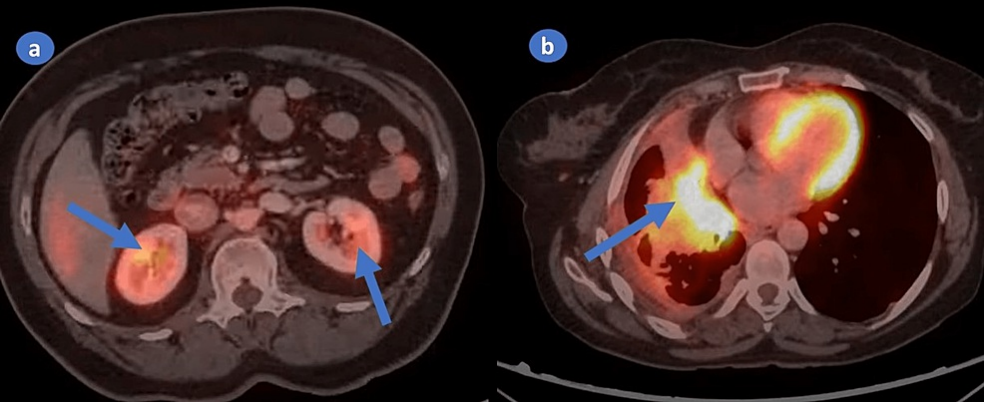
PET-CT revealing enhanced tumor activity in kidneys (a) and lungs (b). PET-CT: positron emission tomography-computed tomography.
